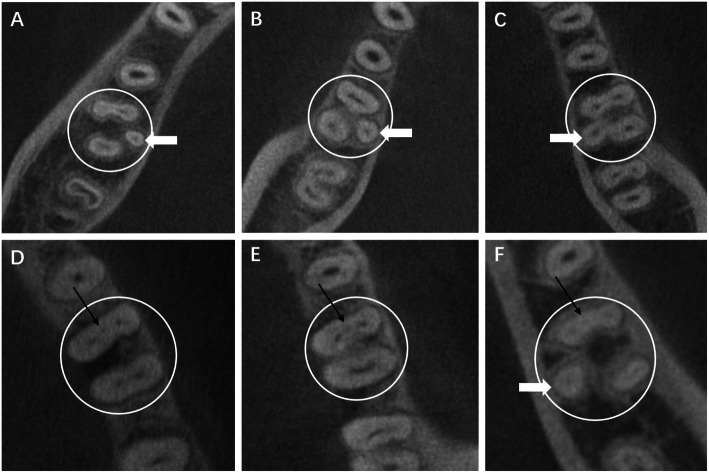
Cases of mandibular first molars with RE or MMC in the axial section; the white circles indicate the examined tooth; the white arrows indicate REs; the black arrows indicate MMCs. CBCT images show (A-C) mandibular first molars with RE, (D-E) mandibular first molars with MMC, (F) mandibular first molars with both RE and MMC
